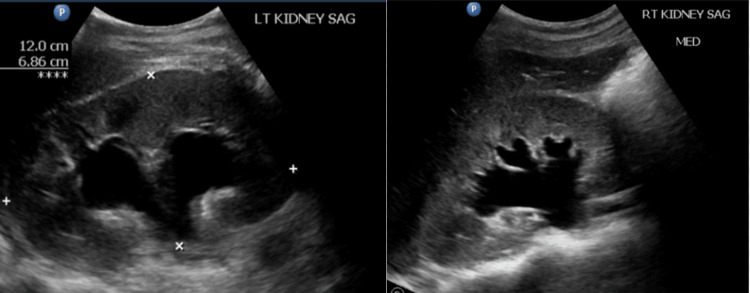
Renal ultrasound revealing severe bilateral hydronephrosis
